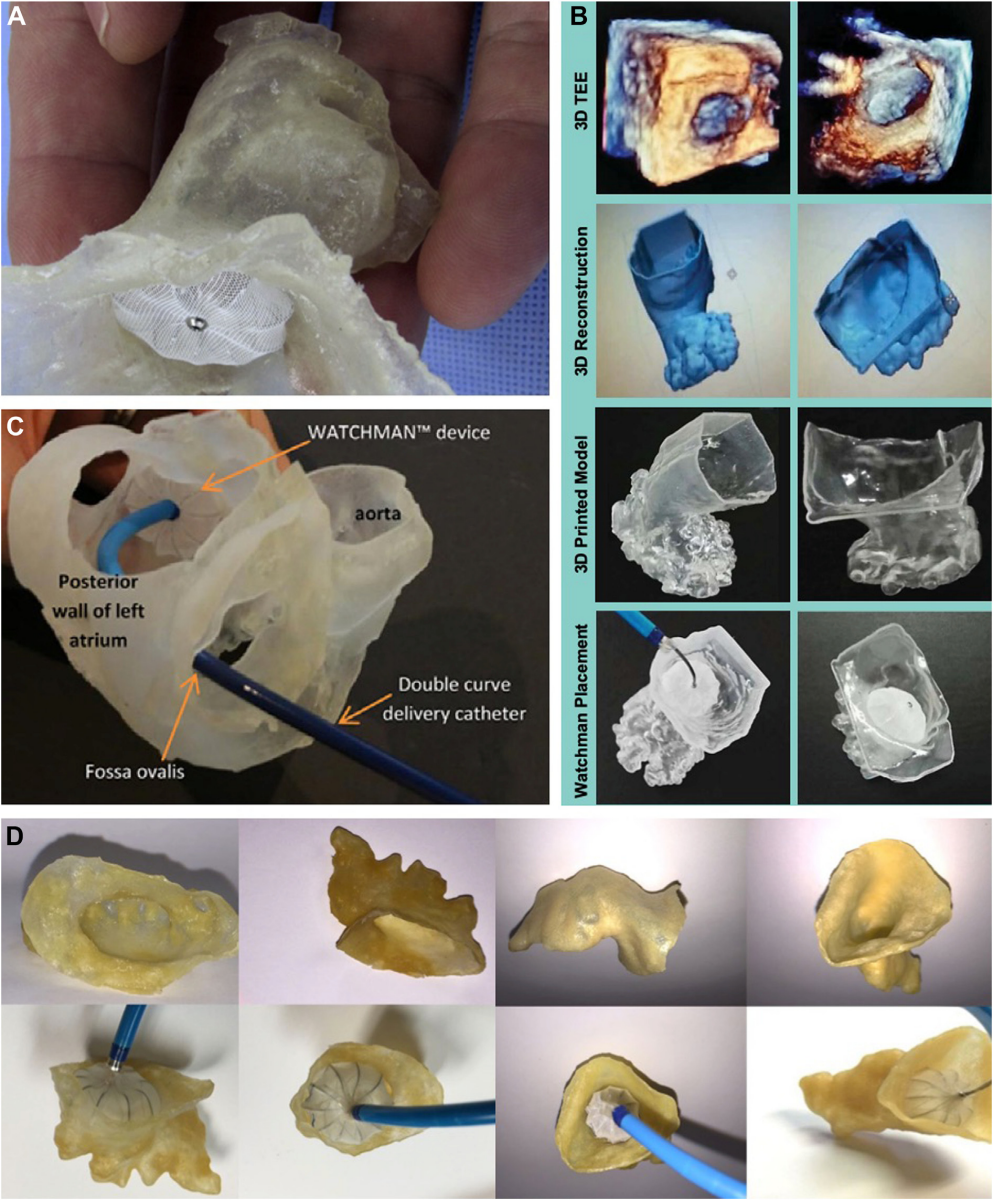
The use of 3-dimensional (3D) printed models in left atrial appendage occlusion. (A) The Watchman device placed within a flexible 3D-printed model demonstrates the clinical utility of 3D printing for device sizing and avoiding procedural complications. (B) 3D-printed models of the left atrial appendage using real-time 3D transesophageal echocardiographic data for assistance with physician planning and decision making. (C) 3D-printed model of a patient’s specific left atrial and left atrial appendage anatomy for assistance in the bench-test selection of catheter curvature for device implantation. (D) Computed tomography-based 3D-printed models for preprocedural planning in left atrial appendage device closure. Adapted from Otton et al,^109^ 2015; Liu et al,^110^ 2016; Wang et al,^69^ 2016; and Obasare et al,^111^ 2017.
